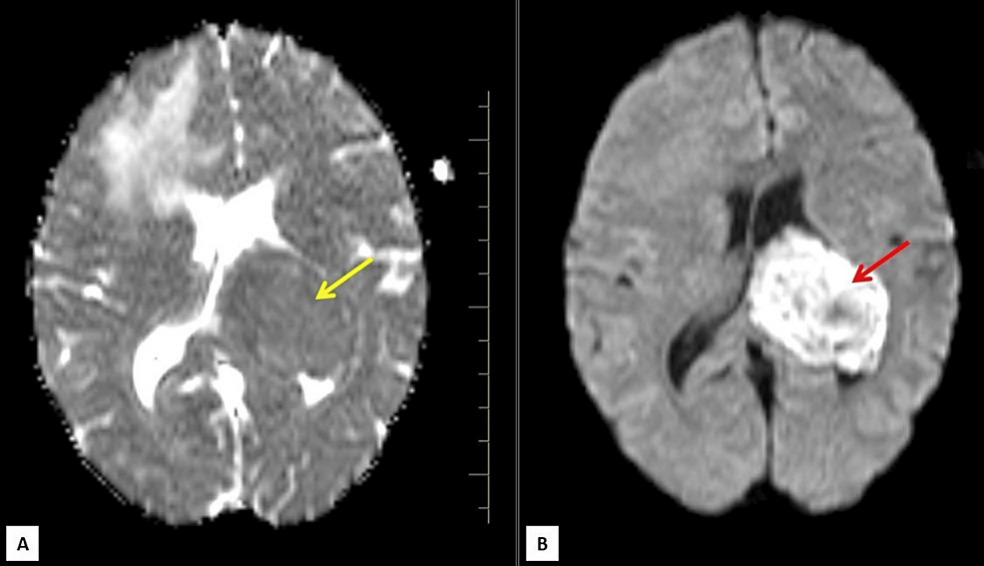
Axial ADC (A) and DWI (B) MRIs show high signal intensity (red arrow) with no obvious ADC hypointensity (yellow arrow) in the intraventricular lesion ADC: apparent diffusion coefficient; DWI: diffusion-weighted imaging
